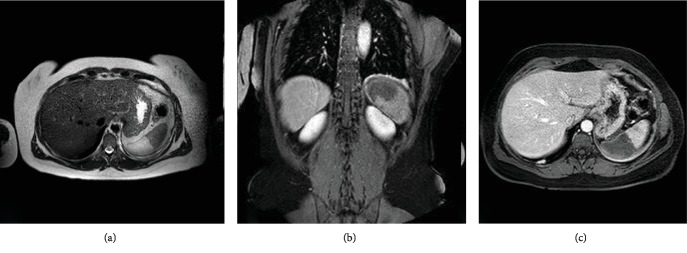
Abdominal MRI. Axial T2-weighted image (a) showing a high-intensity signal in the upper splenic portion. Coronal (b) and axial (c) T1-weighted fat suppressed postcontrast image revealing a hypovascularized area in the corresponding area.
